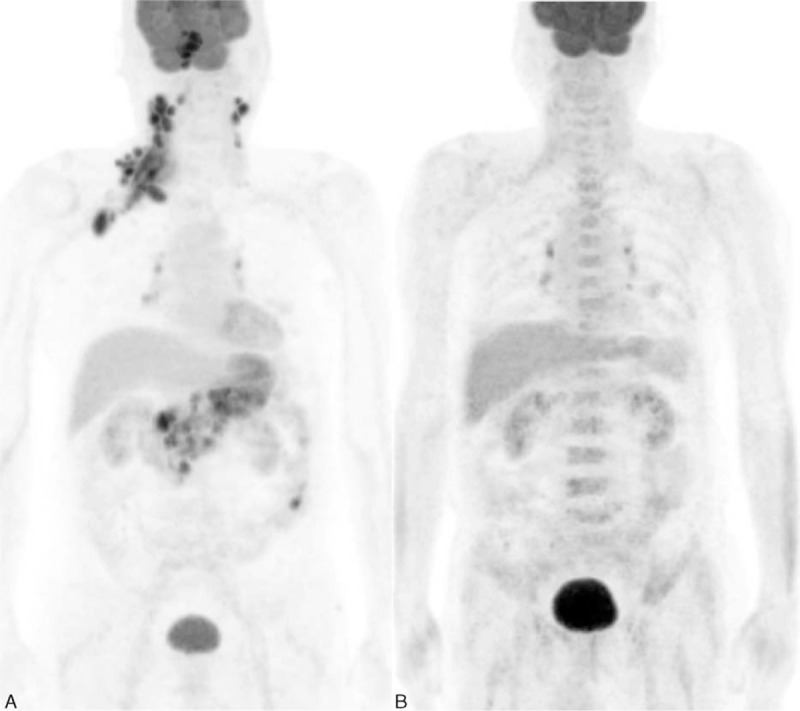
Representative case of a patient with total lesion glycolysis50(<2000). An 83-year-old patient was diagnosed Ann Arbor stage III diffuse large B-cell lymphoma. Serum lactate dehydrogenase was 390 IU/L. A, The patient had lymphoma involvement in the nasal cavity, and total lesion glycolysis50 was measured as 1142.9 on ^18^F-FDG PET/CT. B, Lesions disappeared on follow-up PET/CT, without central nervous system relapse.
